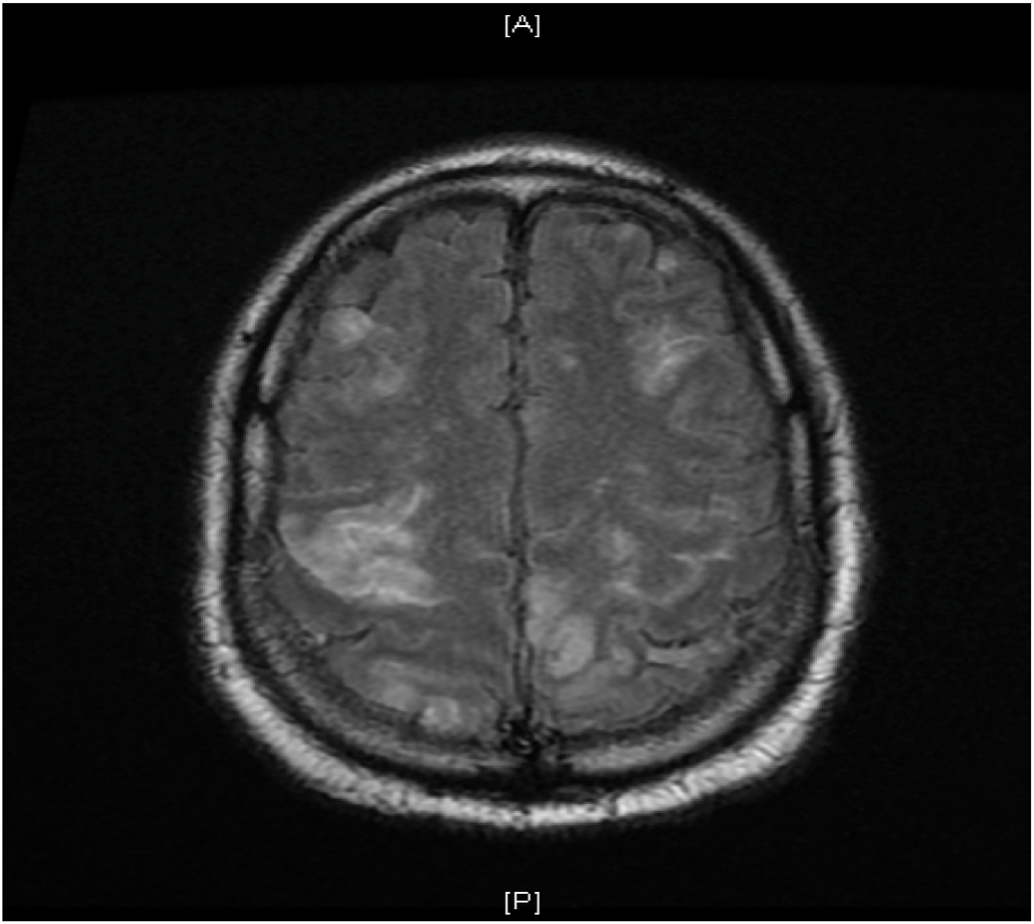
MRI Brain (T2 FLAIR) with multifocal embolic infarcts.
